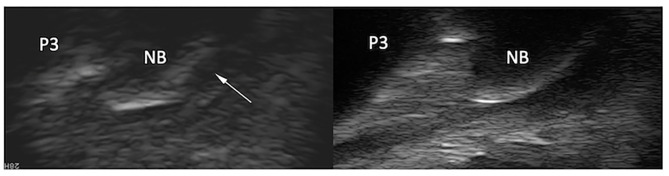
Longitudinal transcuneal ultrasonographic image of the same front foot than Figure 4 (left) and comparative image of a normal navicular bone (right). Only the distal aspect of the navicular bones is visible on the ultrasonographic images. The images have been flipped vertically to obtain the same orientation than the MR images. The erosive defect is characterized by disruption of the normal hyperechoic bone profile and deep penetrating echoes (arrow).
